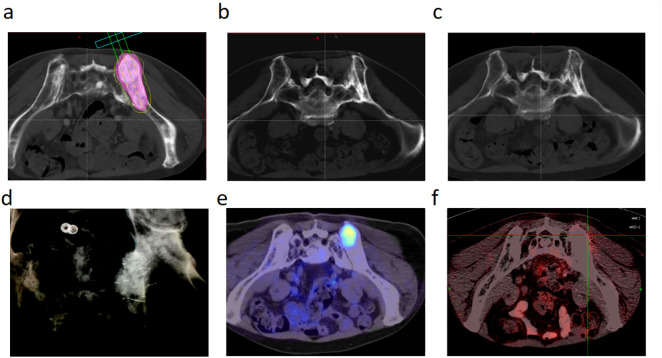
A patient with pelvic metastasis of prostate cancer. (a) Preoperative plan of seed implantation designed with enhanced CT according to ^125^I seed implantation system. (b) Imaging after template-assisted CT-guided needle insertion showed that the needle insertion angle and the depth were good, and no significant displacement of the puncture needle was noted during the intervention. (c) Review immediately after the intervention; CT showed uniform division of seeds in the area of the lesion. (d) Re-examination 4 days after intervention confirmed that the seeds were well located within the lesion. (e) Preoperative PET-CT showed right pelvic metastasis and radiopharmaceutical aggregation in the metastatic lesion. (f) PET-CT 2 years after radioactive ^125^I seed brachytherapy showed no radiopharmaceutical aggregation in the right pelvic metastatic lesion.
